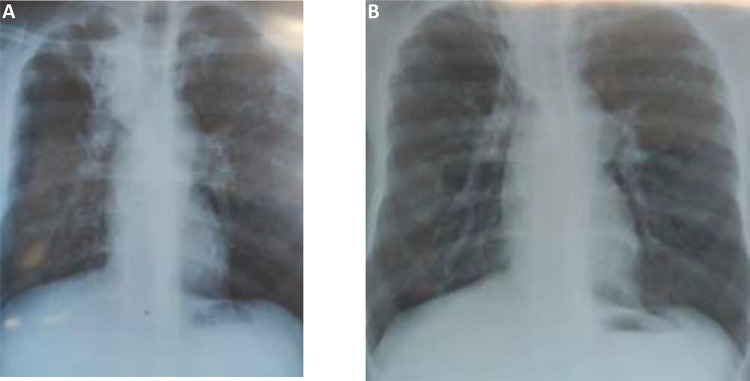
Plain film chest radiographs for patient one - Postero-Anterior view: (A) June 2020, showing bilateral upper lobe infiltration and right upper lobe cavity and right paratracheal hilar lymphadenopathy, (B) October 2020, showing bilateral upper lobe fibrosis and a residual cavity in left upper lobe.
